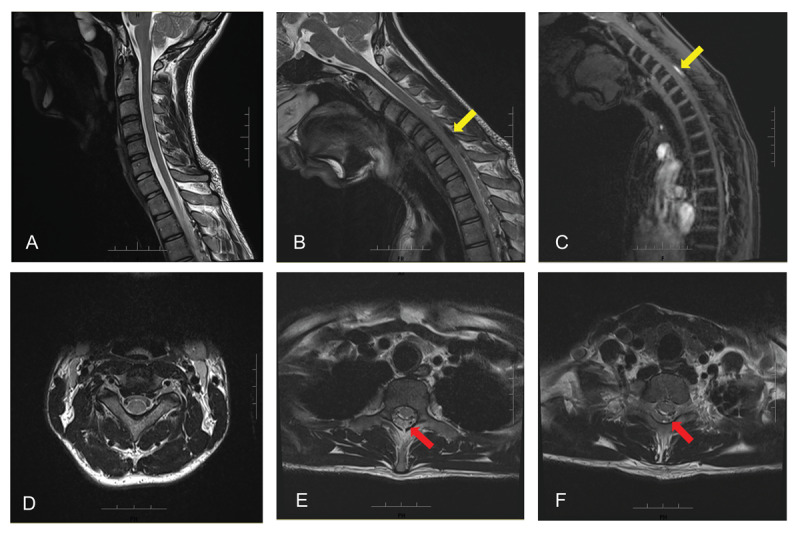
Cervical spinal MRI on neutral and flexion position. (A) Normal unenhanced sagittal T2-weighted imaging on neutral position. (B) Unenhanced sagittal T2-weighted imaging shows flow voids (yellow arrows) in the widened posterior epidural space on flexion position. (C) The flow voids signals were hyperintense on T2 fat-saturated imaging. (D) Normal unenhanced axial T2-weighted imaging on neutral position. (E–F) Axial T2-weighted imaging shows flow voids (red arrows) in the widened posterior epidural space on flexion position from C6 to T3 levels.
